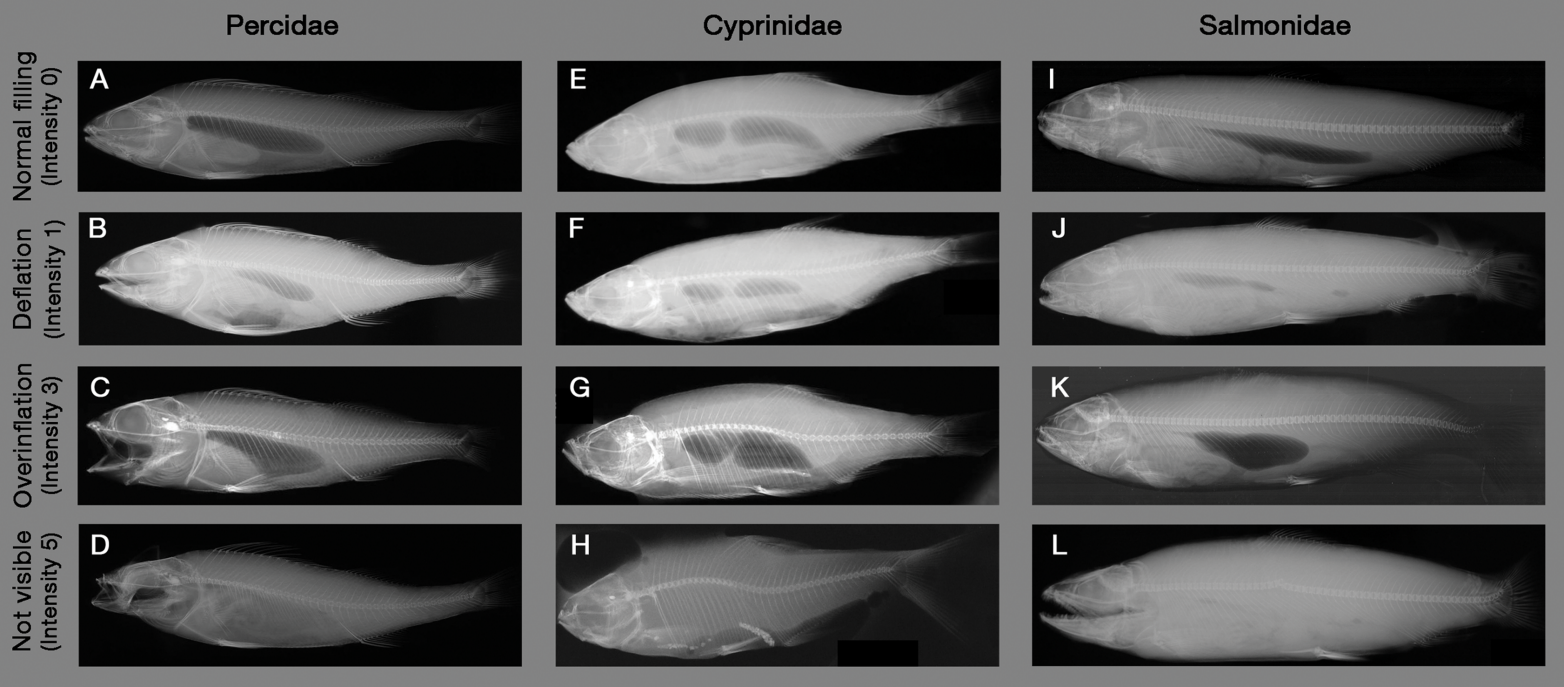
Example X-ray images of swim bladder anomalies in Cyprinids, Salmonids and Percids. (A, E and I) Normal swim bladder filling in Percidae, Cyprinidae and Salmonidae. (B–D) Different levels of swim bladder anomalies in Percidae, (F–H) different levels of swim bladder anomalies in Cyprinidae, (J–L) different levels of swim bladder anomalies in Cyprinidae. Source: Institute of Anatomy, Faculty of Medicine, LMU Munich, Munich, Bavaria, Germany.
